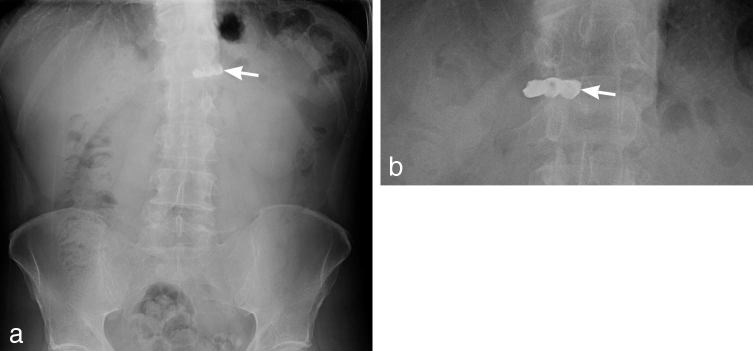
Abdominal anteroposterior radiographs (a, b) demonstrating minimal change in position of a three-unit bridge (white arrows) located in the area of the gastric antrum. The bridge is composed of three crown components attached together.
